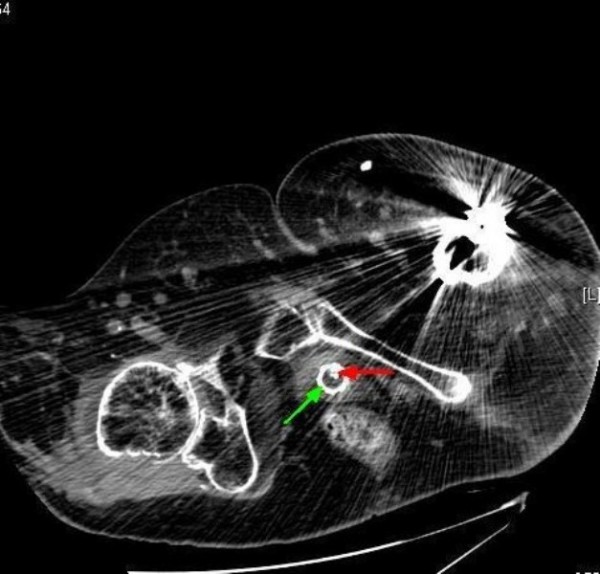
Computed tomography of pelvis (24 February 2009): axial section shows circular ring of stent located just behind symphysis pubis (green arrow); a wire is protruding into the lumen (red arrow).
